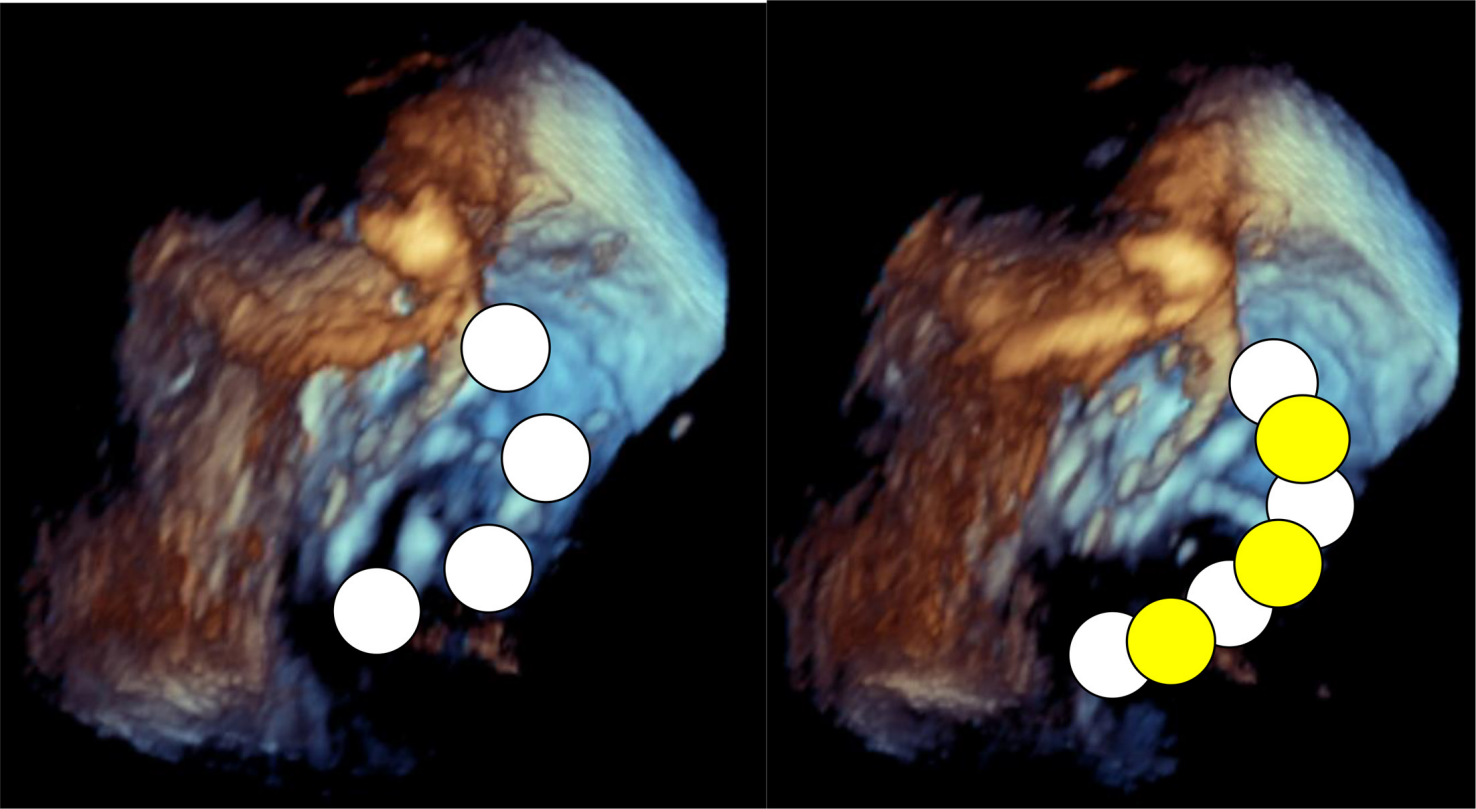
3D TEE-guided PFA catheter rotation to overlap the targeted ablation area. The left side shows the lesion of the previous PFA with white dots, and the right side shows the lesion of the next PFA with yellow dots. See the movie in the online supplemental video 7. PFA, pulsed-field ablation; TEE, transoesophageal echocardiography.
